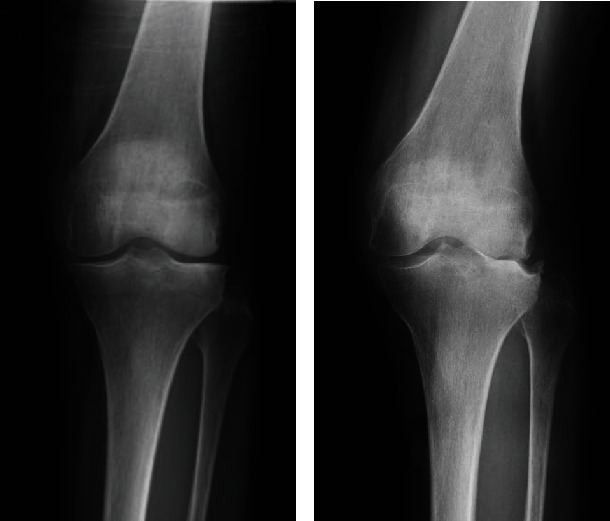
Preoperative radiographs of the left knee. (a) Radiograph at the first visit to our hospital shows Kellgren-Lawrence grade II and mild osteoarthritis of the lateral joint surface. (b) Radiograph at 1 month later shows Kellgren-Lawrence classification grade IV and severe valgus osteoarthritis.
